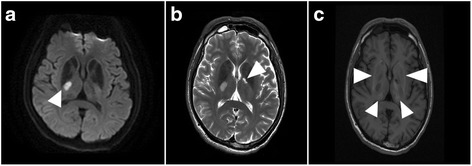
The findings of brain MRI. a Acute ischemic infarction involving the right posterior limb of internal capsule presented as hyperintense area in diffusion weighted images (*arrowhead*). b An old lacunar infarction involving the left genu of the internal capsule was seen in T2 weighted images (*arrowhead*). c Nonenhanced T1 weighted images showed hyperintense signal in bilateral basal ganglia, thalami (*arrowheads*) and cerebellar dentate nuclei (not shown)
